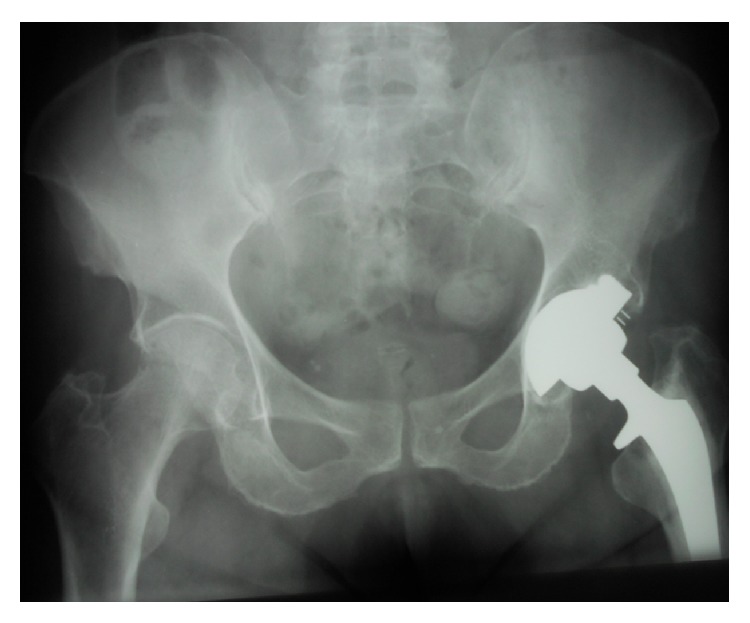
Anterior-posterior hip X-rays in patient number 4 after 13 years on Coumadin. The right hip is unchanged, Ficat stage II.
